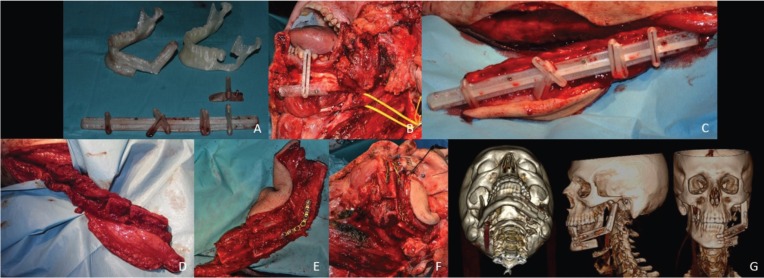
A - Design and manufacture of the surgical guides for the mandibular osteotomy and the fibula graft for the mandibular reconstruction. B - Positioning and intraoperative fixation of the surgical guide for the mandibular osteotomy. C - Positioning and intraoperative fixation of the surgical fibula osteotomy guide. D - Multiple osteotomies of 2-3 cm in the osteomyocutaneous fibula graft. E - Preparation and fixation of the segments, 2 to 3 cm, for transport and subsequent mandibular reconstruction. F - Mandibular reconstruction previously prepared with free fibula graft segments. G - Postoperative 3-D computed tomography of the mandibular reconstruction with a fibula graft.
